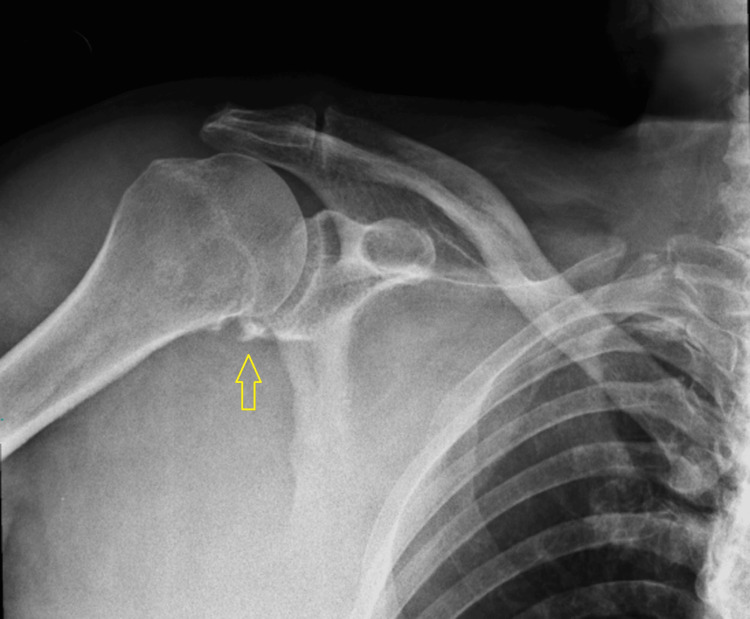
Anteroposterior X-ray of the right shoulder displaying the fracture of the right scapular glenoid (indicated by the yellow arrow).
